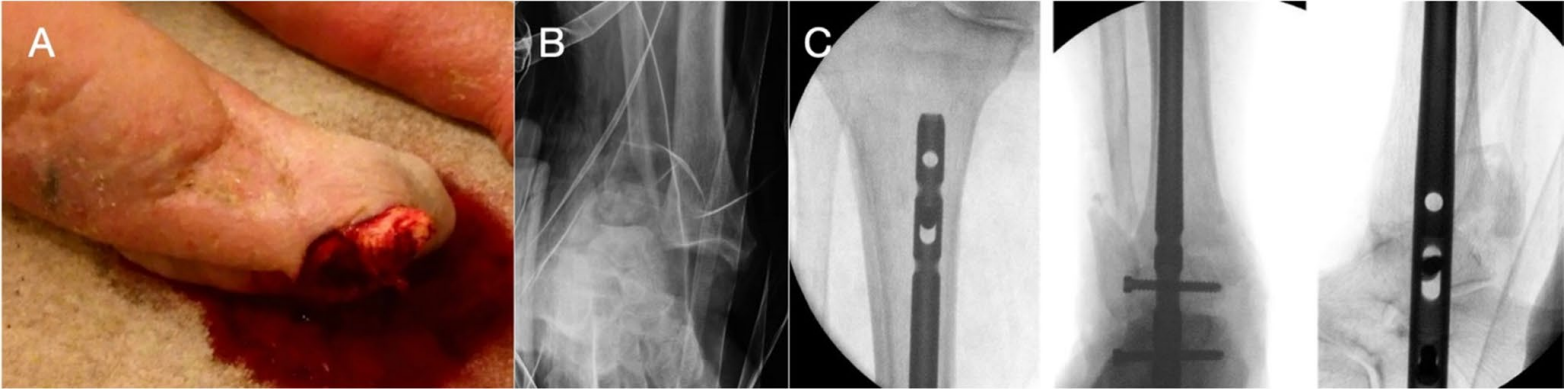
Picture taken by ambulance crew (A) at time of injury showing extruding tibia out of medial skin, with foot folded up behind leg. (B) shows trauma radiograph of dislocated ankle and (C) intraoperative imaging of a retrograde femoral nail (Stryker T2) being used to span the zone of injury. Tibia shortened to allow primary closure of medial skin
